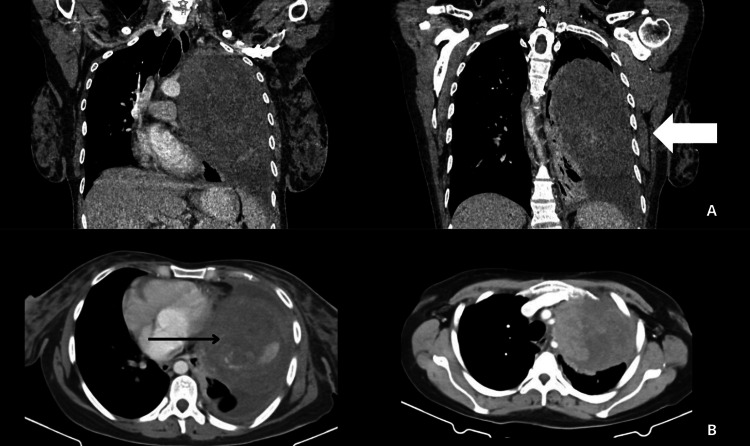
Computed tomography scan of the chest A: The white thick arrow shows the pulmonary mass lesion in a coronal section view. The lesion has an approximate longitudinal dimension of 16.8 cm. B: The black thin arrow shows the lesion in a cross section view. The lesion has an approximate dimension of 11.02 cm anteroposterior and 10.3 cm transverse. This is an expansive lesion of the left lung that infiltrates the visceral and parietal pleura, in close contact with the following structures: pericardium, subclavian artery, and ipsilateral aortic arch. The lesion exerts a mass effect with contralateral mediastinal displacement.
